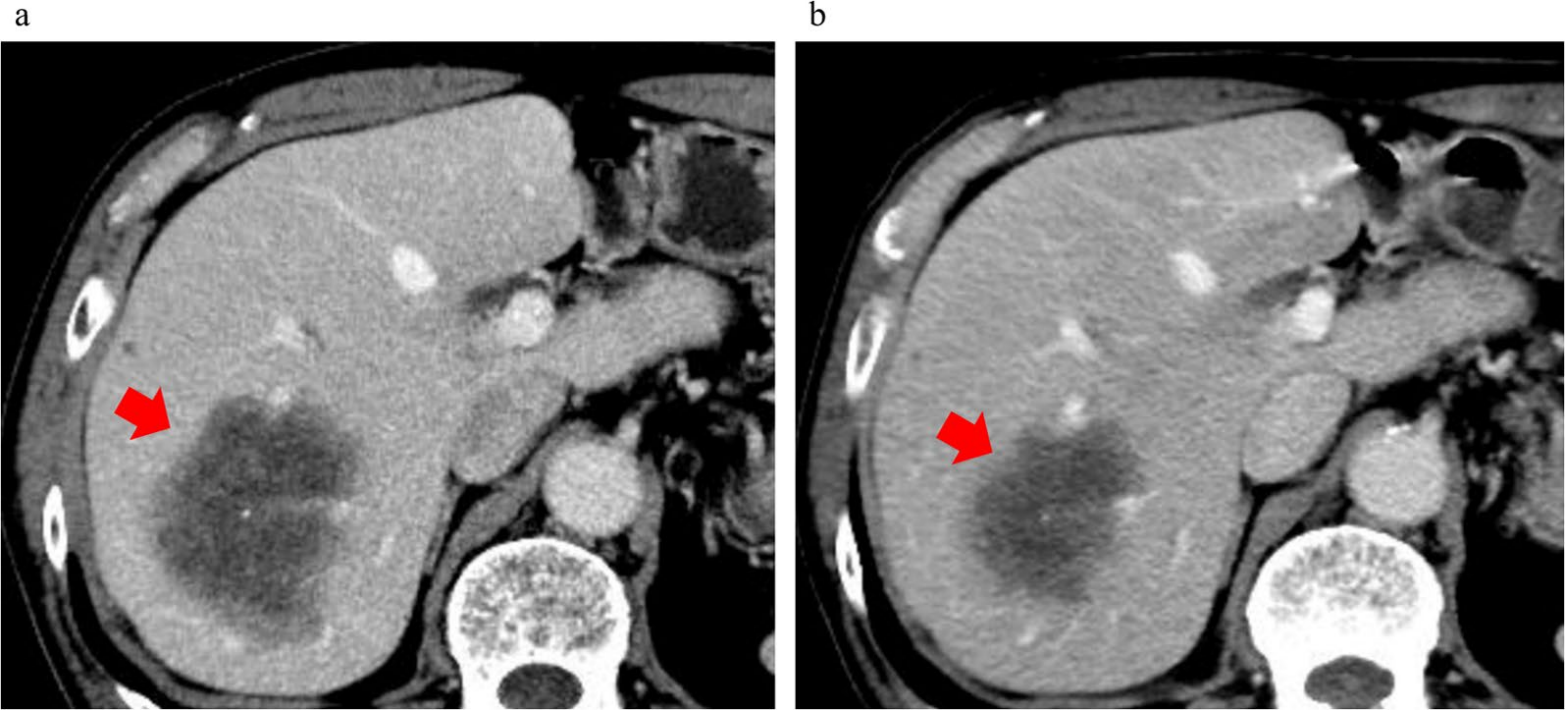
a Image findings of contrast-enhanced dynamic-CT of Case 2 before NAC. There was a mass of 75 mm in diameter with low density in the central part and a faint ring-enhancement effect in the S7/8 of the liver (red arrow). b After NAC, the tumor shrank (red arrow)
